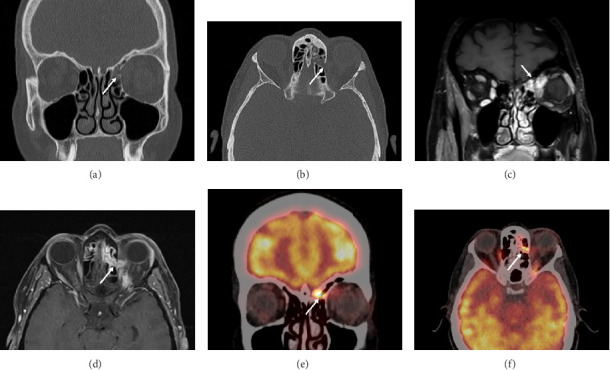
Imaging studies of a patient with a cT4bN0M0 mucosal melanoma of the ethmoid sinus: (a) coronal CT image; (b) axial CT image; (c) coronal postcontrast T1-weighted MR image with fat saturation (T1 MR Gd FS); (d) axial T1 MR Gd FS image; (e) coronal 18F-FDG PET/CT; (f) axial coronal 18F-FDG PET/CT; white arrows point toward the primary tumor.
